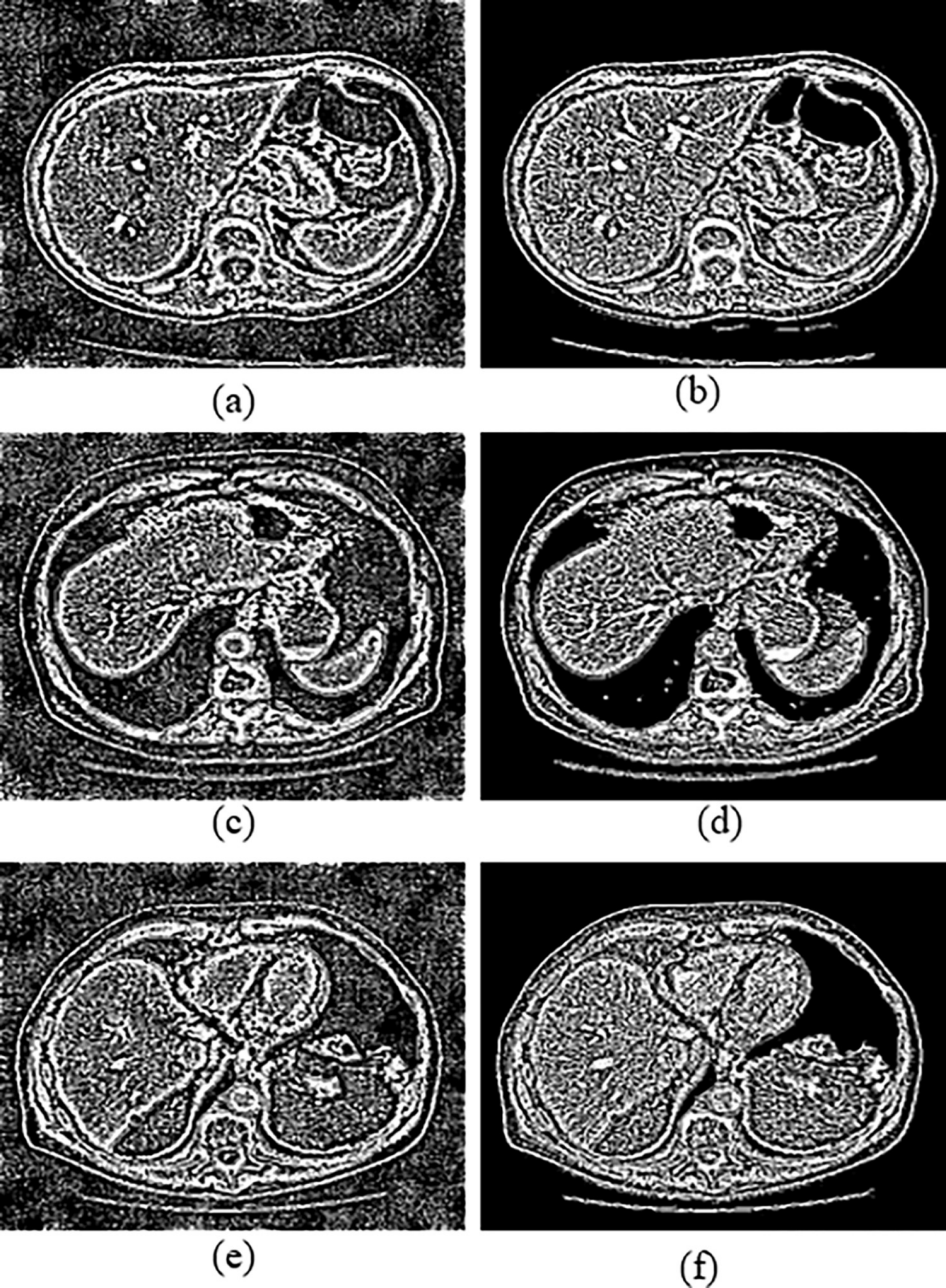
Residual images: (a, c, e) represents low-dose CT images and (b, d, f) represents corresponding denoised images of the proposed method on L035, L129 and L250.
